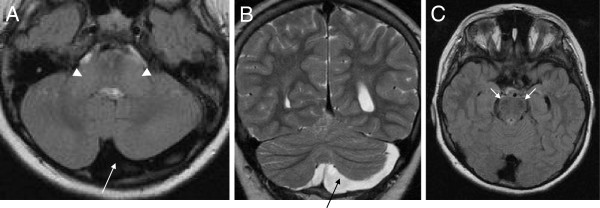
Developmental asymmetry of cerebellar hemispheres. Axial T1 (A) and coronal T2 (B) MRI images of patient #4 demonstrating developmental asymmetry of the cerebellar hemispheres (long arrows) without cerebellar atrophy. Apart from this finding, this patient shows the common infratentorial signs of ARSACS: thickening of the middle cerebellar peduncles (arrow head, A) and linear hypointensities of the mid-pons (best seen on axial FLAIR, arrows, C).
